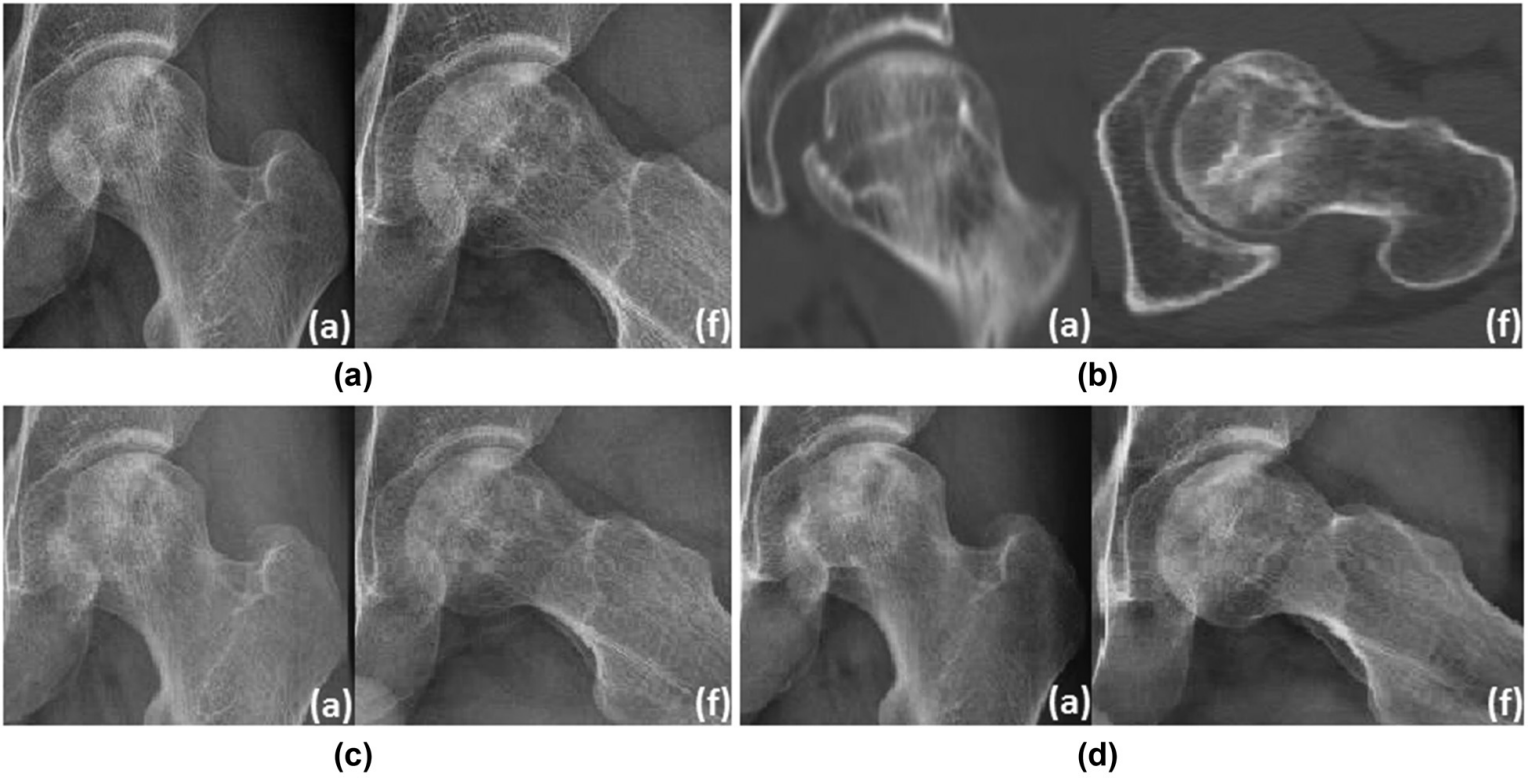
Typical case of ONFH without cystic area: (a) (radiograph imaging at an anteroposterior (a) and frog (f) lateral view indicated extensive necrosis of the left femoral head), (b) CT showed no obvious cystic change area, (c) 6 months follow-up, and (d) 1.5 years follow-up.
